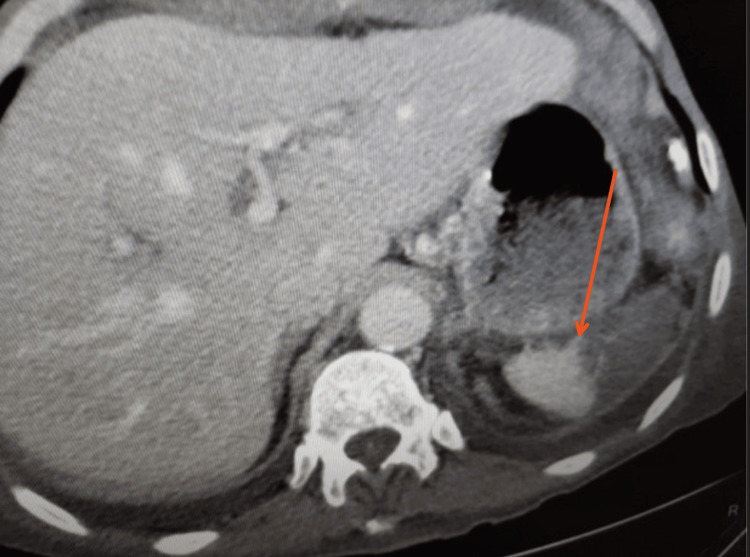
CT scan showing a splenic infarct (red arrow) following the Warshaw procedure, which resolved three months post-surgery.
